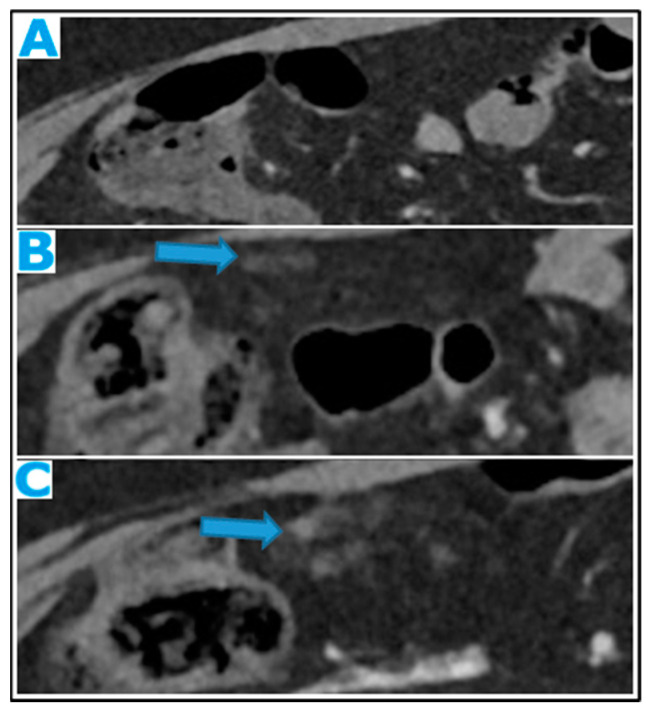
Axial CE-CT (A) from one year prior (disease-free peritoneum); (B) from four months prior; (C) current follow-up. Note the early stages and evolution of omental deposits (arrows).
